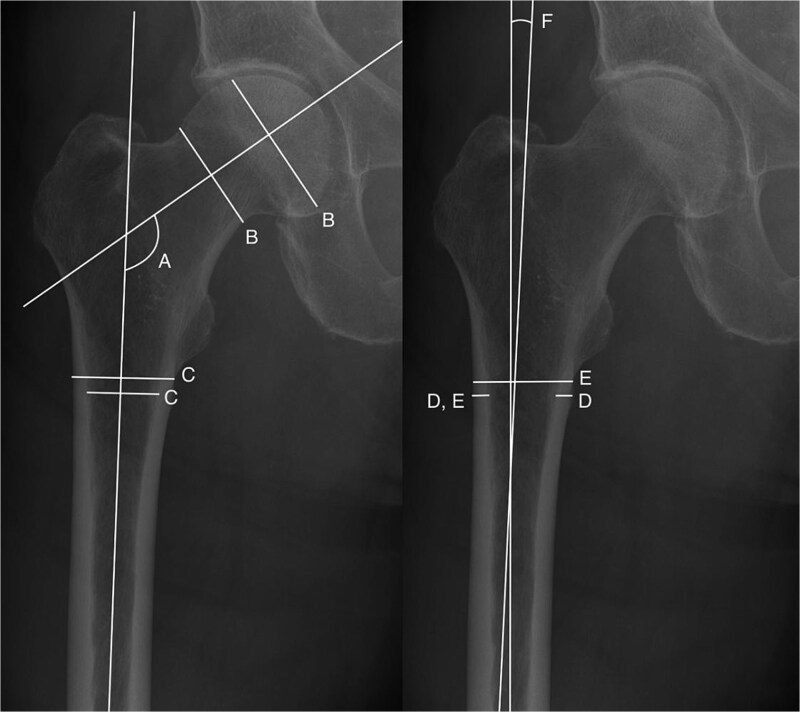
Assessment of femoral geometry on a 2-dimensional anteroposterior radiograph of a femur on the contralateral side relative to the fracture. A = Femoral neck-shaft angle. B = Head-neck offset ratio. C = Total cortical thickness index (CTi). D = Lateral to medial cortical thickness ratio. E = Lateral cortical thickness index (LCTi). F = Lateral femoral bowing.
